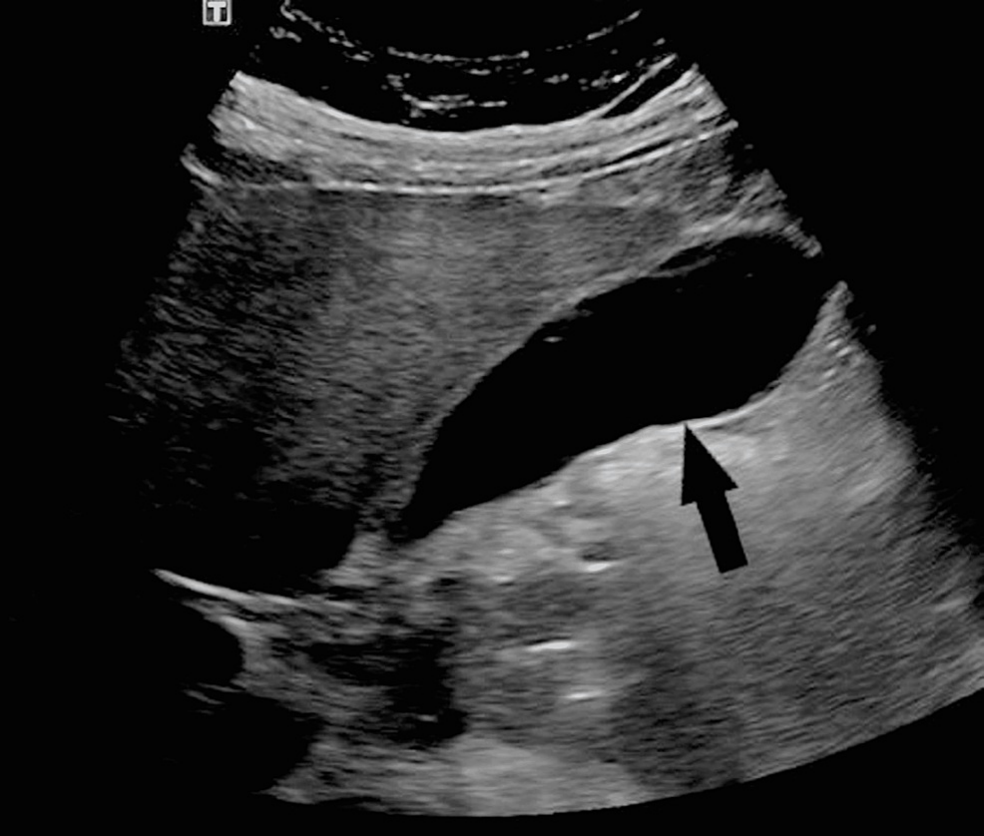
Ultrasound abdomen shows no gallstones.
